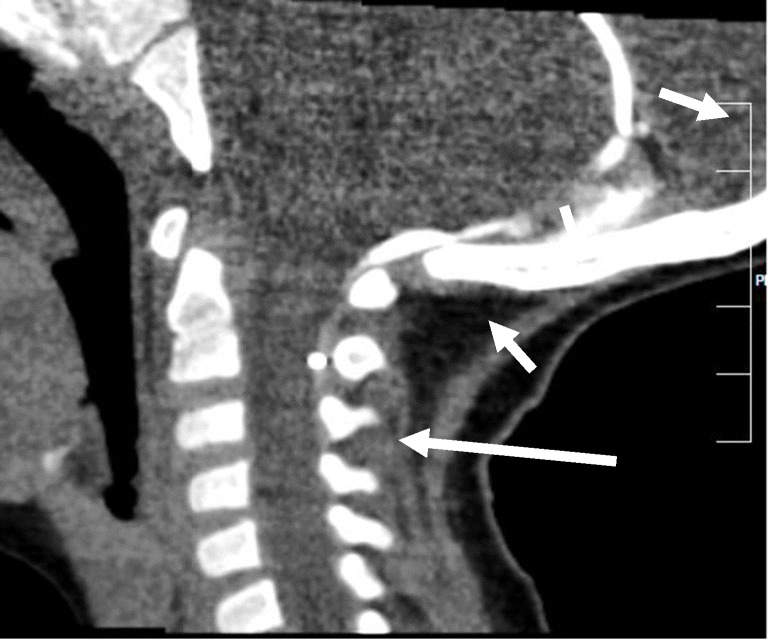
Case 5, spiral CT of the skull base and the upper cervical spine after contrast medium application via the side port of the baclofen pump: The tube system was intact up to the intraspinal entry of the tube at the level of L4/L5, without evidence of leakage. Contrast medium distribution presents epidurally dorsal to the CSF space at C2 (long arrow), pushing between the dural sheets of the tentorium (short arrow). There is no evidence of intrathecal contrast medium
